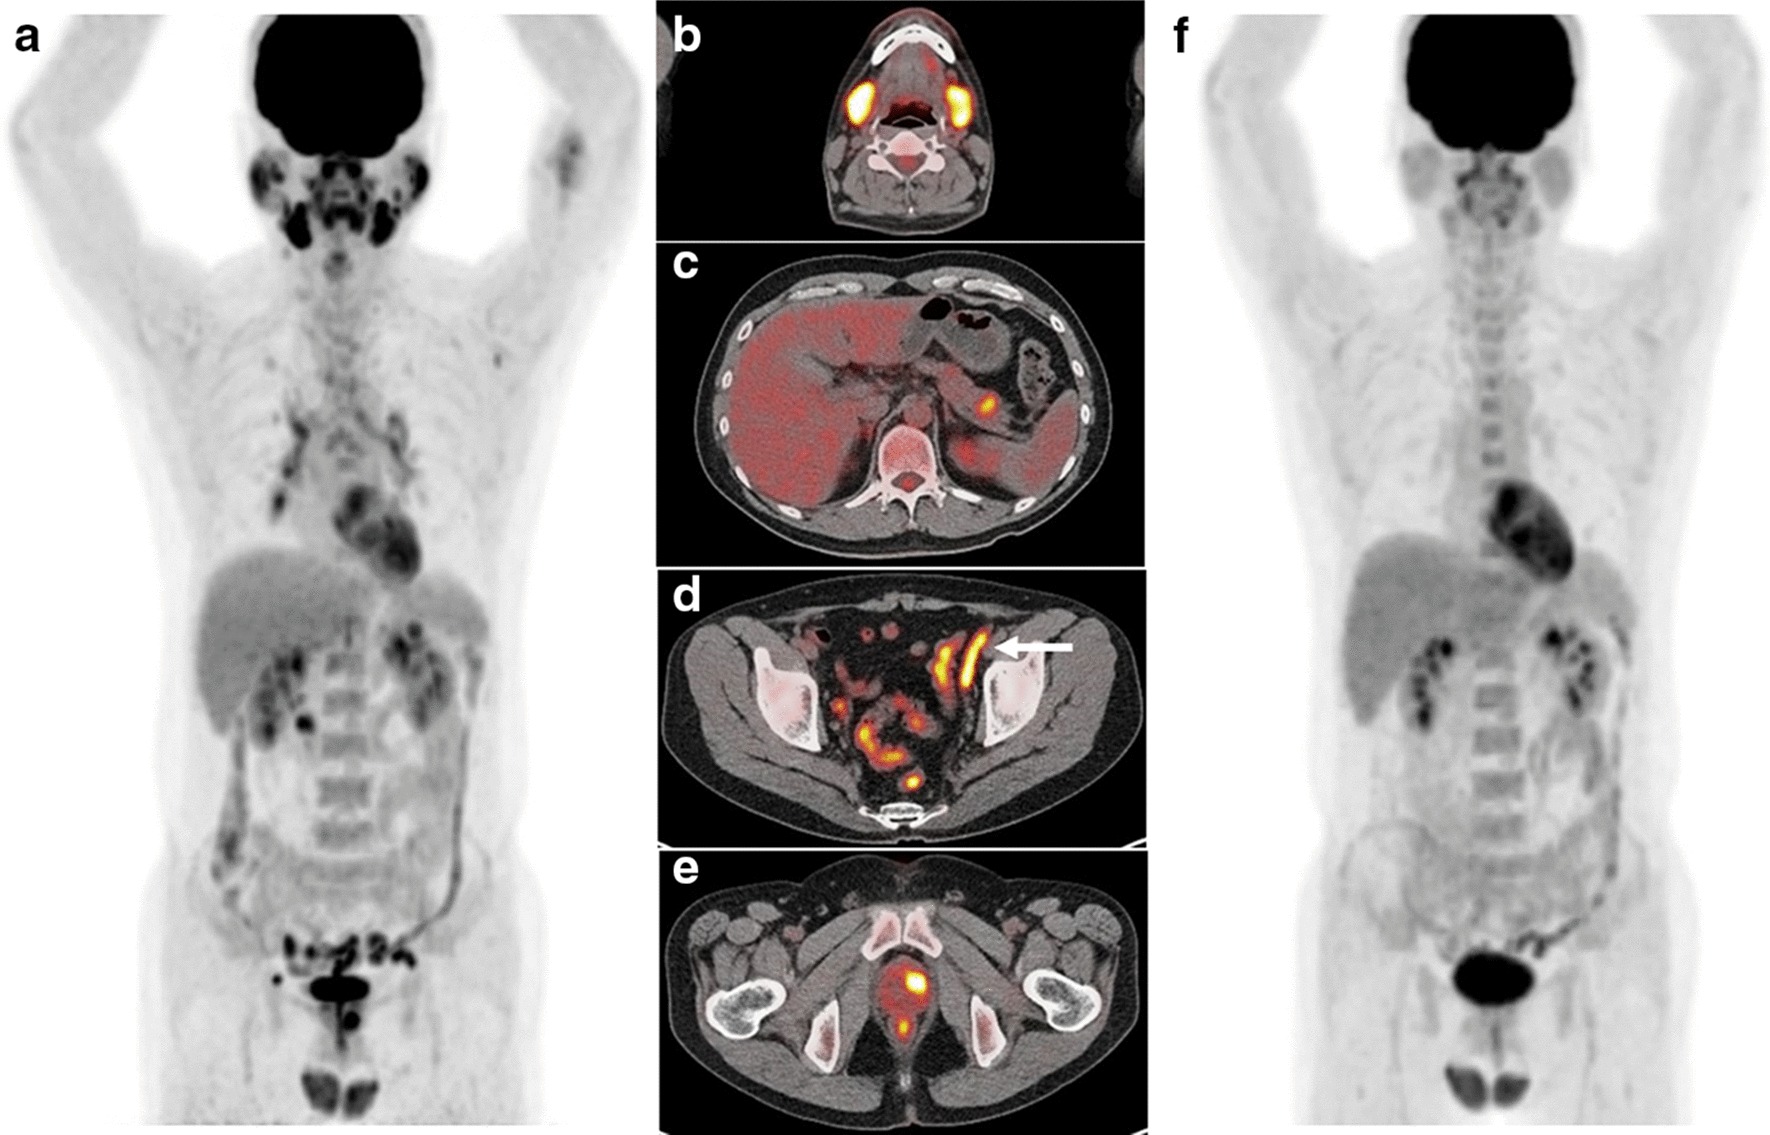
Pretreatment [^18^F]FDG PET maximum intensity projection (MIP) image (a) and transverse fused PET/CT images b–e of a 45-year old male with IgG4-related disease shows multiple organ involvement, including of salivary glands, cervical lymph nodes, pancreas (head and tail), spermatic cord (arrow), and prostate. Thirty-six days after initiation of corticosteroid therapy, [^18^F]FDG PET MIP image f shows complete disappearance of the abnormal [^18^F]FDG uptake
